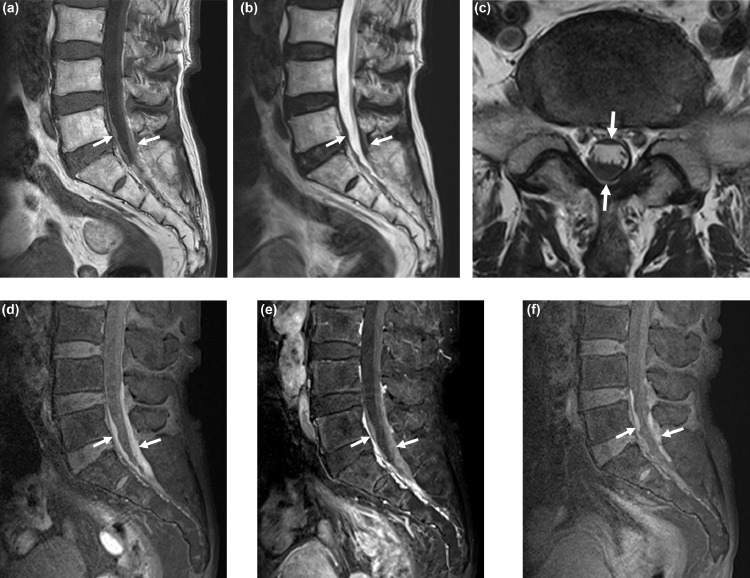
An 83-year-old man with subacute spinal subdural hematoma. (A and B) The sagittal T1-weighted (A) and T2-weighted (B) images show hyperintensity and hypo-intensity of the subdural hematoma (arrows), respectively. (C) The axial T2-weighted image confirming the subdural location of the hematoma (arrows). (D and E) Sagittal fat-saturated T1-weighted images without (D) and with (E) enhancement show hyperintensity of the subdural hematoma (arrows). The fat-saturated T1-weighted image visualizes the extent of the hematoma more clearly than conventional T1- and T2-weighted images. (F) Sagittal fat-saturated T1-weighted magnetic resonance image at the 1-month follow up shows markedly decreased volume of the hematoma (arrows).
